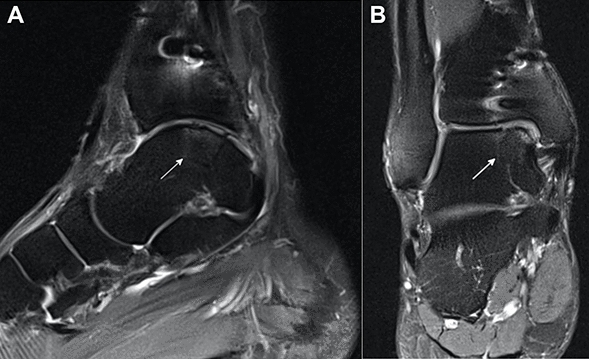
MRI assessment of the replaced cartilage. Sagittal (A) and coronal (B) intermediate-weighted turbo spin echo images of the right ankle of a 26-year-old male patient (at the time of AOT) with a MOCART 2.0 score of 85 points at 19.3-years follow-up. The patient was treated with AOT (1 osteochondral dowel, white arrow) combined with an osteotomy of the medial malleolus. *AOT* Autologous osteochondral transplantation; *MOCART* Magnetic Resonance Observation of Cartilage Repair Tissue
